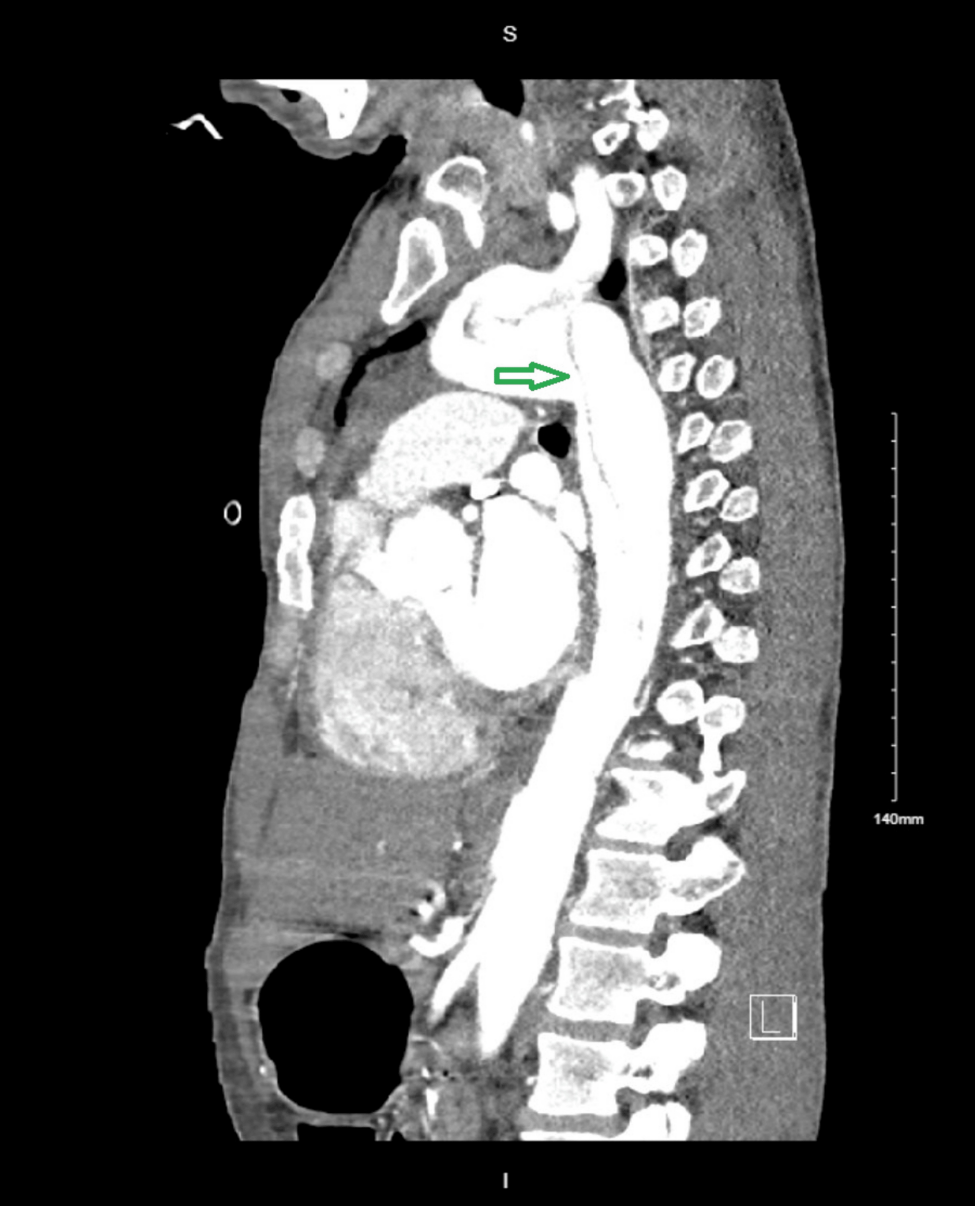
CTA of the chest - sagittal plane Green arrow: acute type A aortic dissection involving the descending aorta CTA: computed tomography angiography
